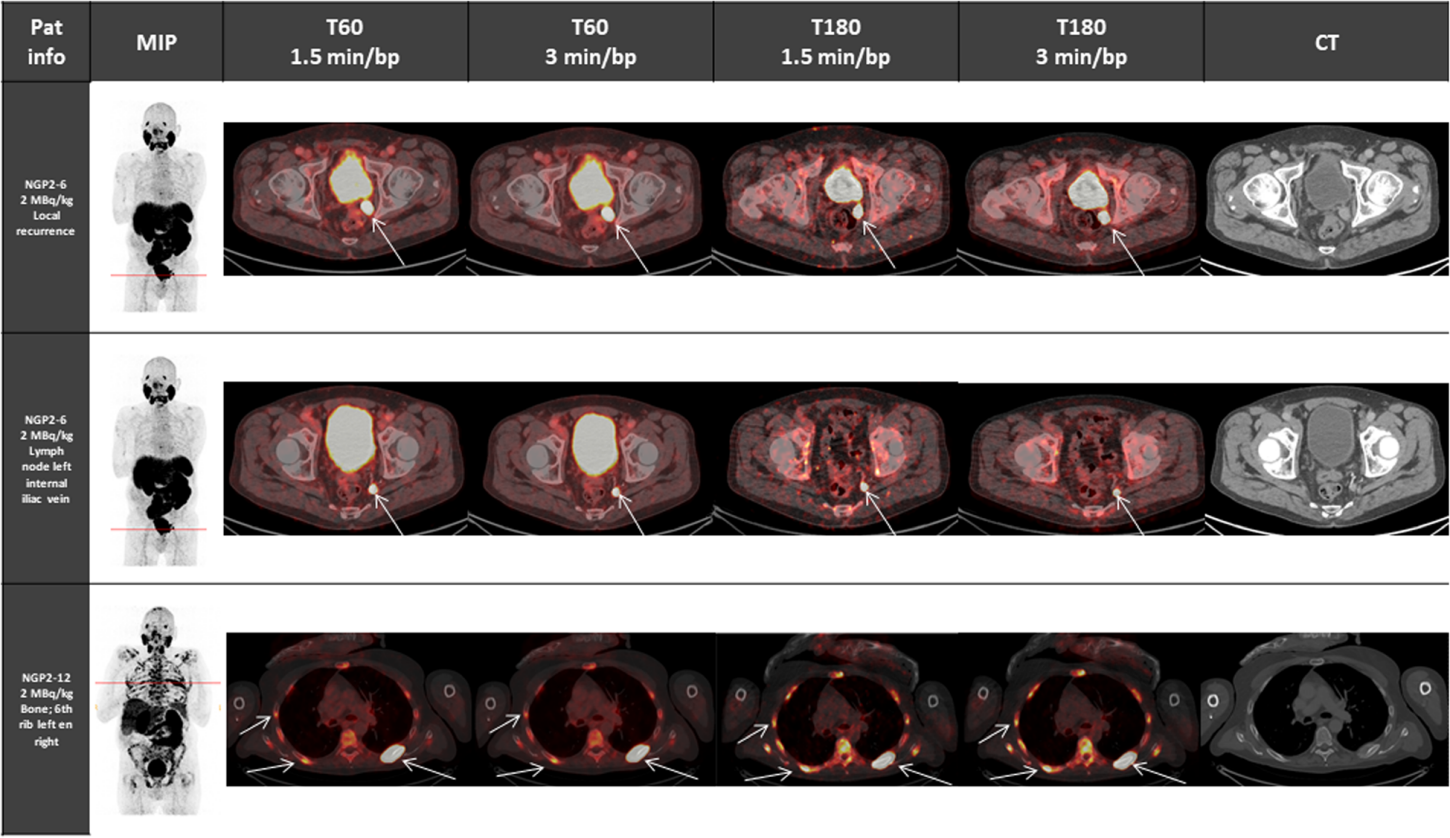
Overview of patient images who were administered 2 MBq/kg who presented with common lesion locations: local recurrence (first row), lymph node metastases (middle row) and bone metastases (last row), each presented as a MIP image (at T60, 3 min/bp) followed by axial images (axis line displayed on MIP image) of all reconstructed time frames (T60, 1.5 and 3 min/bp and T180, 1.5 and 3 min/bp), and the high dose CT image
